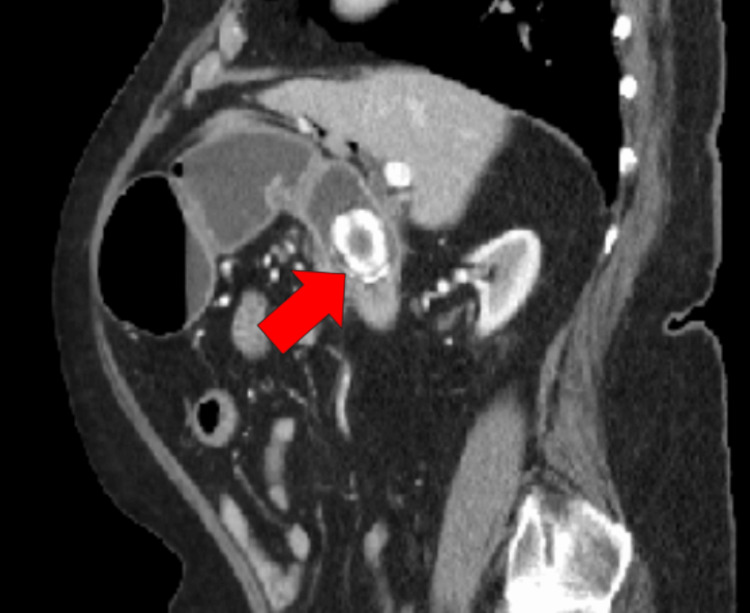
Contrast-enhanced abdominal-pelvic CT image (sagittal view). This image demonstrates dilation in the duodenum secondary to the gallstone. An arrow points to the ectopic gallstone. The cholecystoduodenal fistula is seen between the duodenum and the biliary system. CT: computed tomography.
